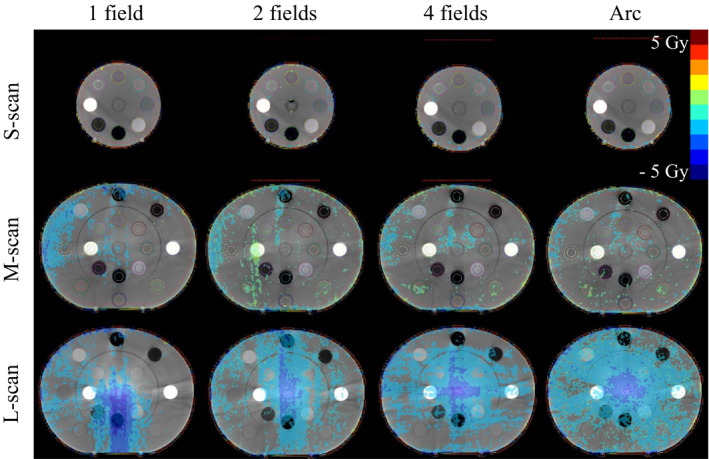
Subtraction image of the planning computed tomography and cone beam computed tomography dose distributions. S‐scans, M‐scans, and L‐scans are shown in the upper, middle, and lower rows, respectively. The irradiation techniques are (from left to right) single field, two parallel opposed fields, 4‐field box, and single arc
